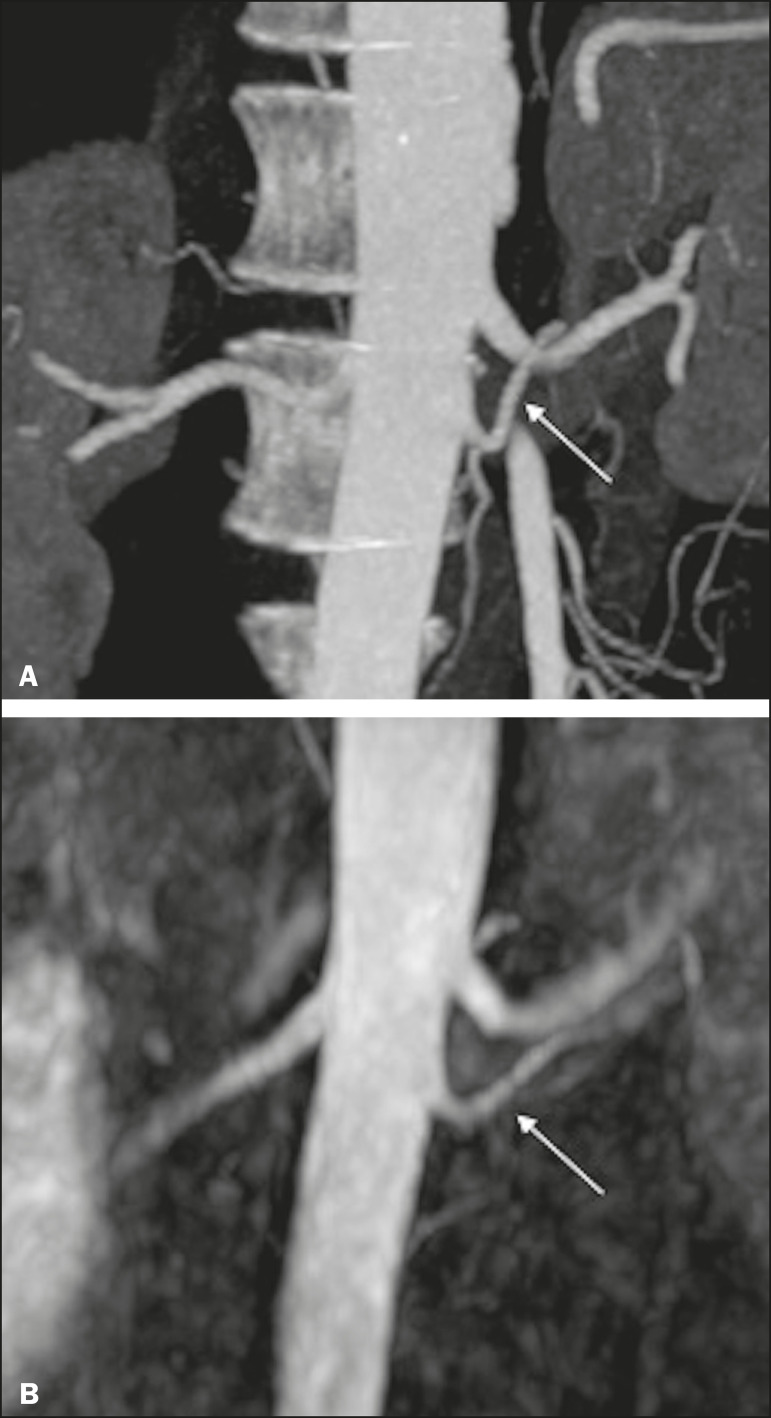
Multiple renal arteries. CTA (A) and unenhanced MRA (B), showing multiple renal arteries on the left (arrows).
